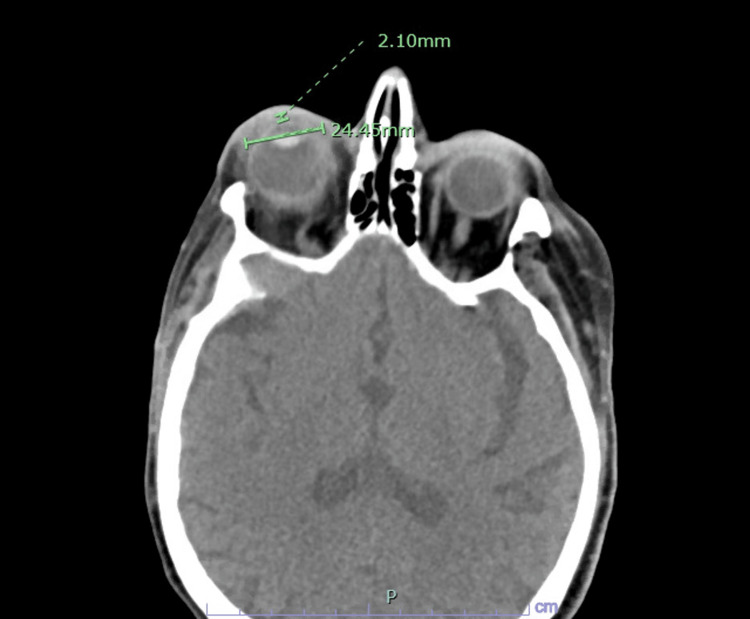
CT of the orbits shows right periorbital/palpebral soft tissue cellulitis with early abscess and diffuse wall thickening of the right optic globe sclera and cornea.
